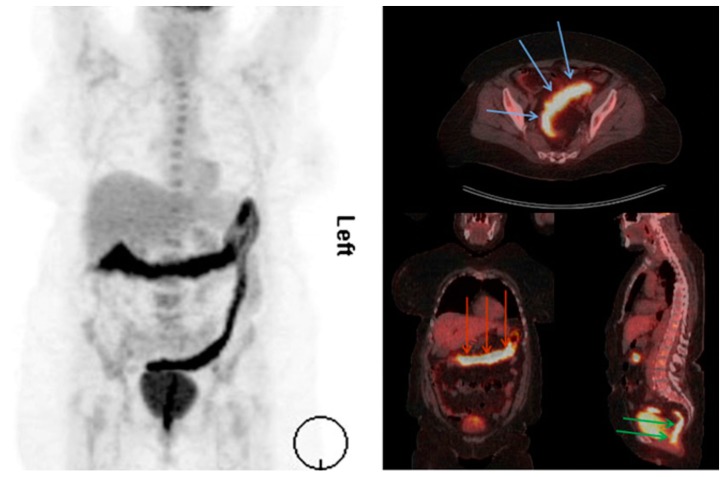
^18^F-FDG PET/CT scan of ulcerative colitis (UC) patient. Extent of disease is seen on the left and in areas indicated by arrows in the right panel [124].
